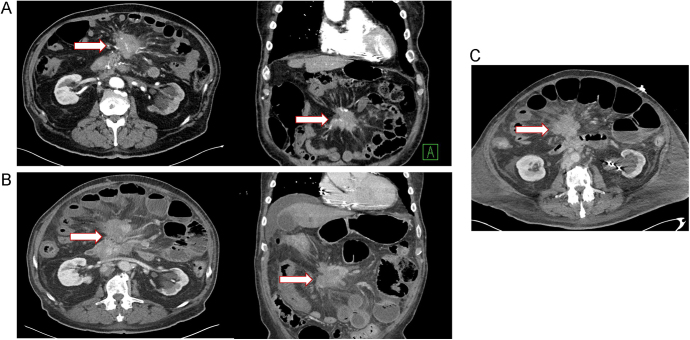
CT scan of patient 1. (A) A mesenteric mass with confluent lymphadenopathy causing encasement and occlusion of the SMV, and severe narrowing of the SMA. (B) CT scan performed after the initial cycle of ^177^Lu-DOTATATE showing interval dilation of the small bowel with some air-fluid levels, and wall thickening of the mid to distal small bowel with pneumatosis, adjacent small foci of free air, and gas within mesenteric veins, likely related to bowel ischemia. (C) Diffuse dilation of the small bowel and pneumatosis intestinalis consistent with ischemic changes, and no focal evidence of obstruction.
